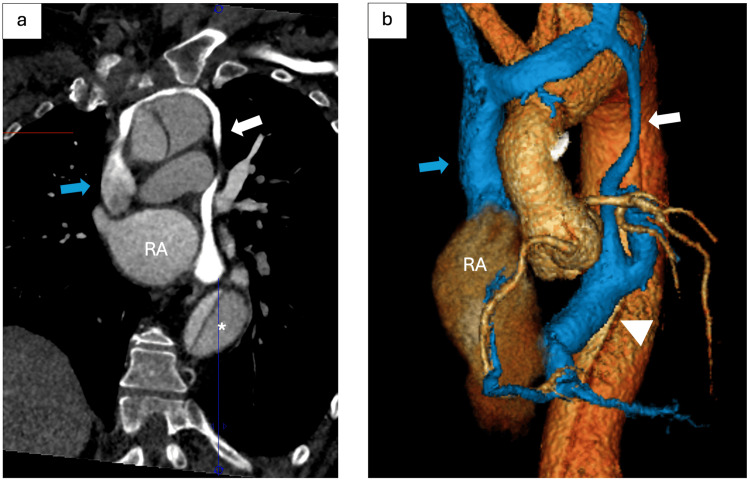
Persistent Left Superior Vena Cava Draining Into the Coronary Sinus on Computed Tomography and Three-Dimensional Reconstruction (a) Curved coronal reconstruction of contrast-enhanced computed tomography of the chest and (b) three-dimensional volume rendering reconstruction of the great vessels demonstrate a persistent left superior vena cava (PLSVC) (white arrow) draining into the coronary sinus (arrowhead). Right SVC (blue arrow) drains normally into the right atrium (RA). Partially visualized dissection flap in the descending aorta (*).
